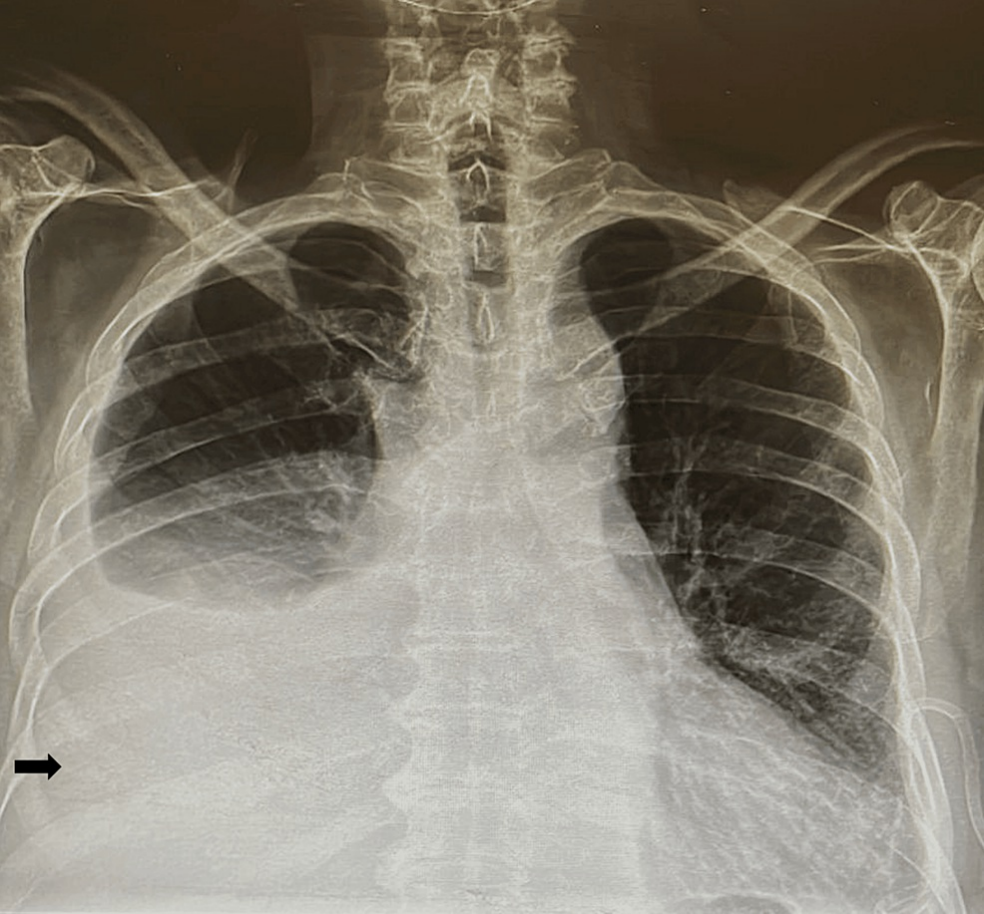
Chest X-ray at admission Upon admission, the chest x-ray revealed the presence of pleural effusion that was bilateral with a right-sided predominance (black arrow).
